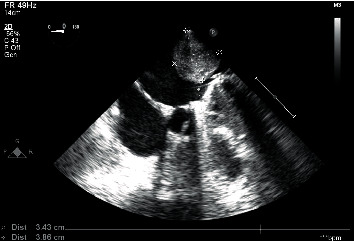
Echocardiography showing a mass in the atrium before surgery.
